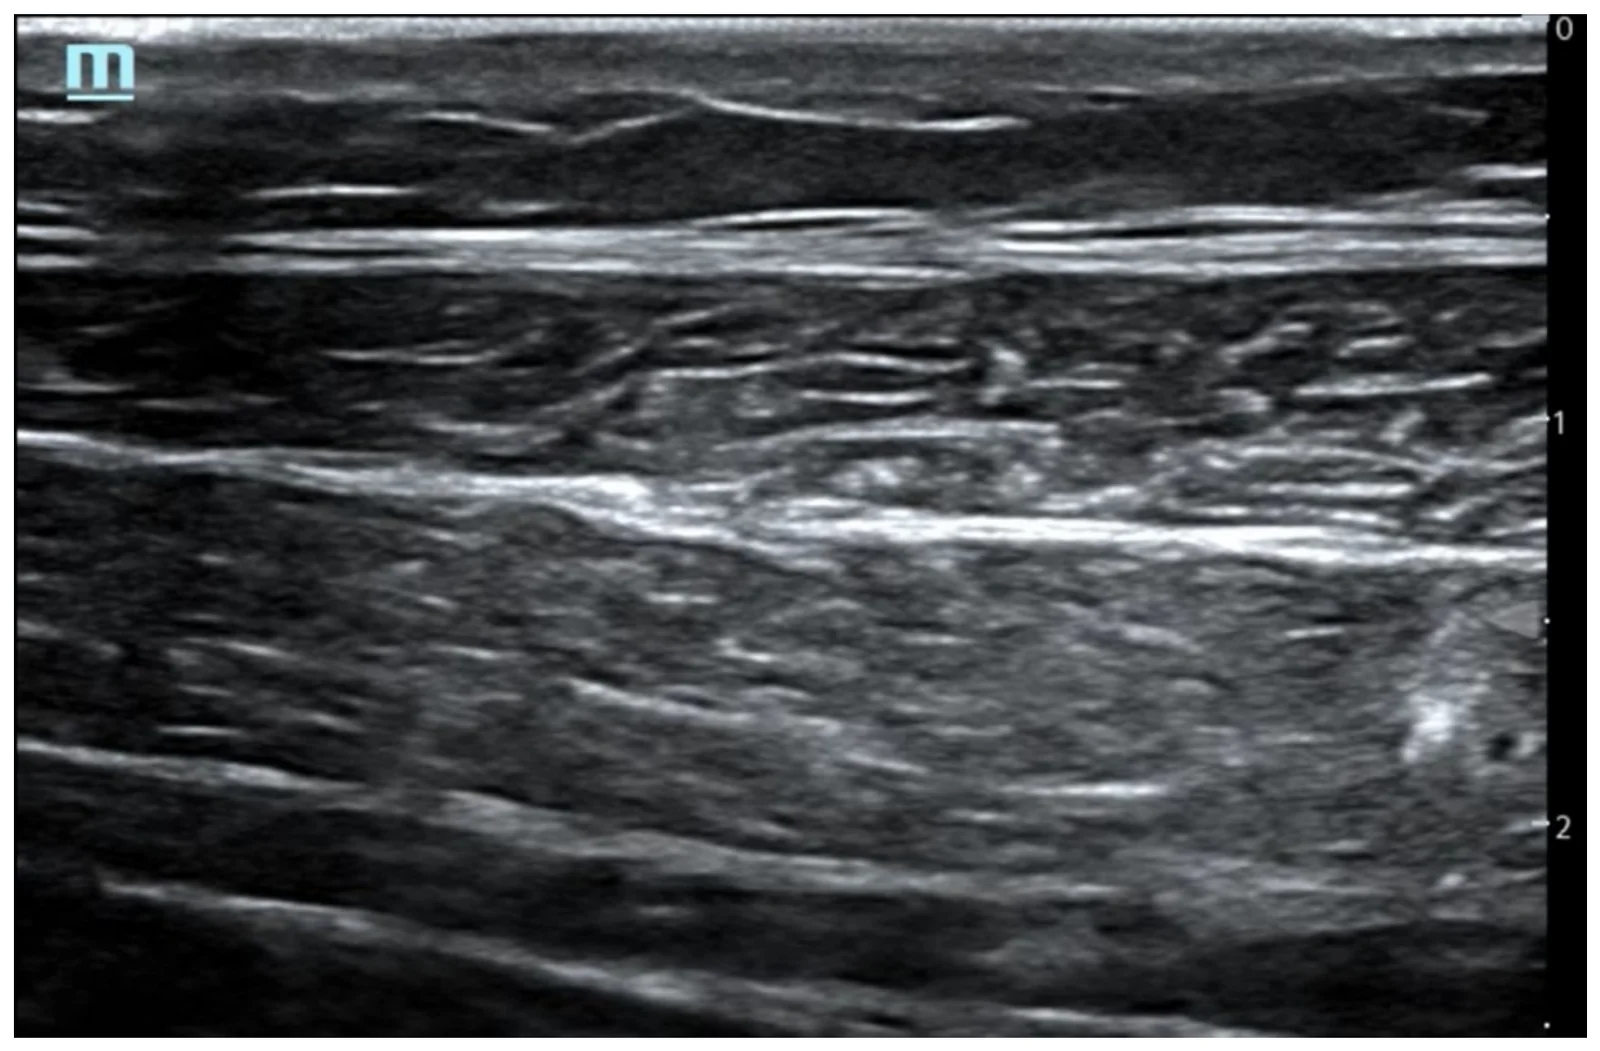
Ultrasound view of external oblique muscle, internal oblique muscle and transversus abdominis muscle in medial axillary line.
